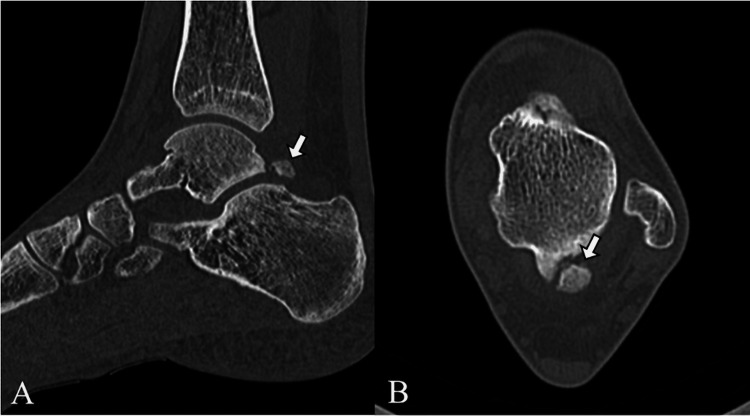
Imaging findings of the os trigonum at initial presentation (computed tomography (CT)) (A) Sagittal view; (B) Axial view. Arrows indicate the os trigonum.
